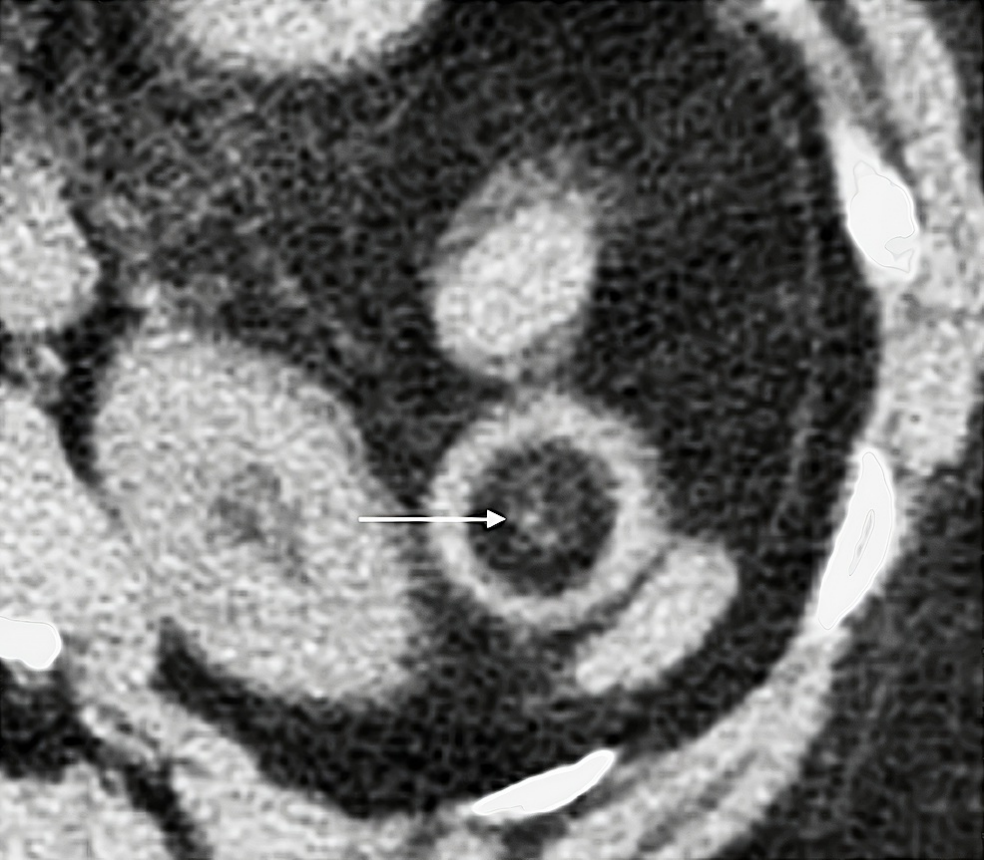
Axial CT through the jejunum (magnified) at the level of the left kidney shows jejunal intraluminal fat density, consistent with mesenteric fat (horizontal arrow)
